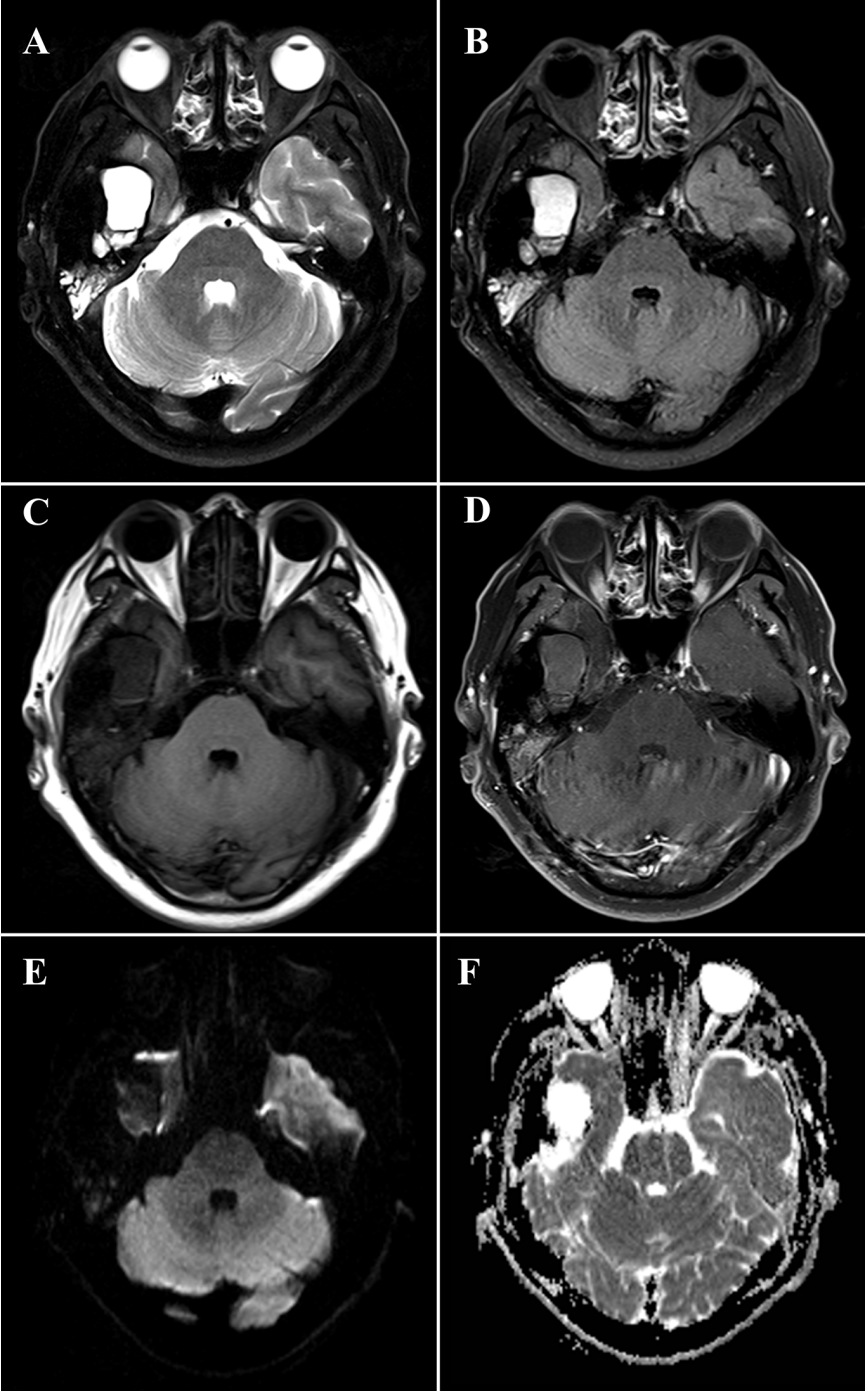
Magnetic resonance imaging (MRI) of a 48-year-old man with diffuse tenosynovial giant cell tumor (D-TSGCT) in right temporomandibular joint (TMJ). MRI reveals a predominantly hypointense lesion with focal cystic changes on T2-weight imaging (A), fluid-attenuated inversion recovery (FLAIR) sequence (B) and T1-weighted imaging (C), which infiltrates the medial cranial fossa. Mild enhancement at the wall of the cystic part and no enhancement of the solid part was observed after administration of contrast agent (D). The lesion is hypointense on diffusion-weighted imaging (DWI) (E) and displays heterogeneous intensity on the apparent diffusion coefficient (ADC) map (F).
